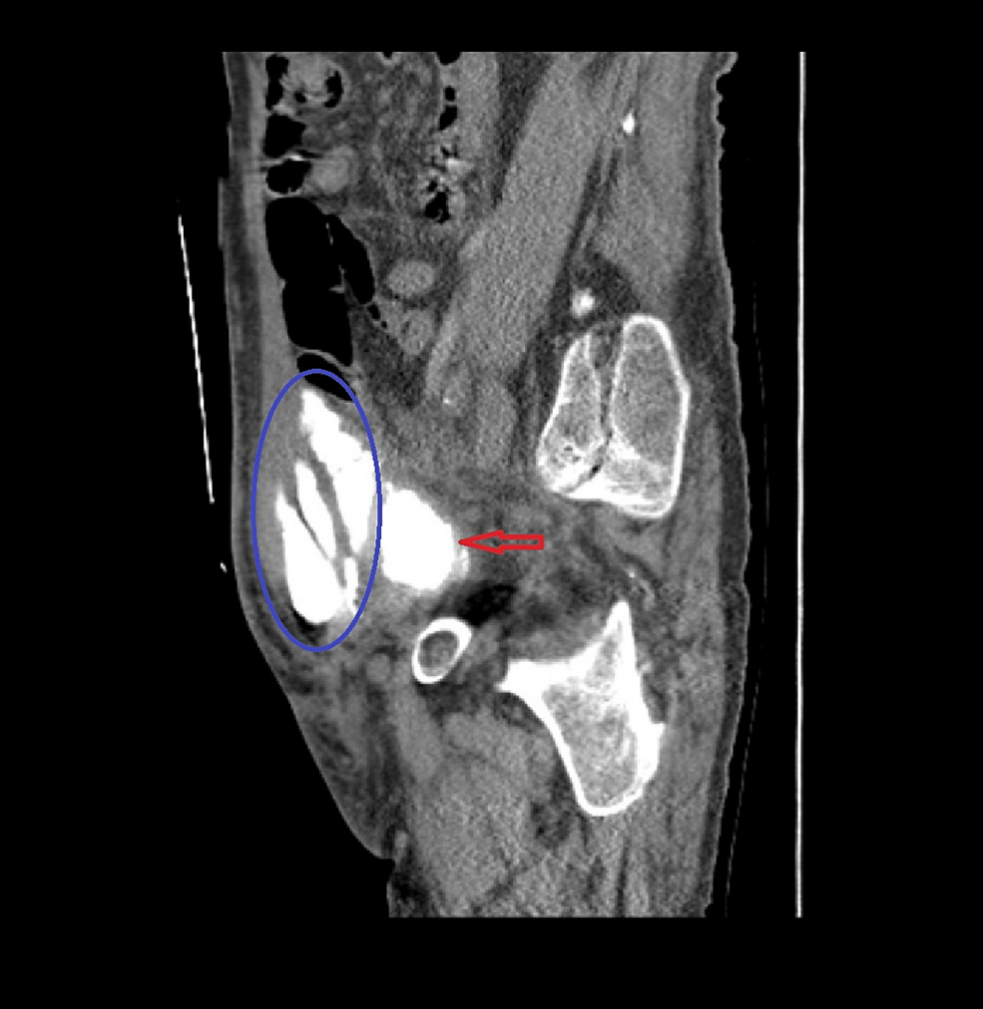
Computed tomography (sagittal view) study shows urinary bladder anterior superior wall large defect (red arrow) measuring about 4.3 × 4 cm communicating with anterior pelvic encysted fluid complicated localized urinoma (blue circle).
